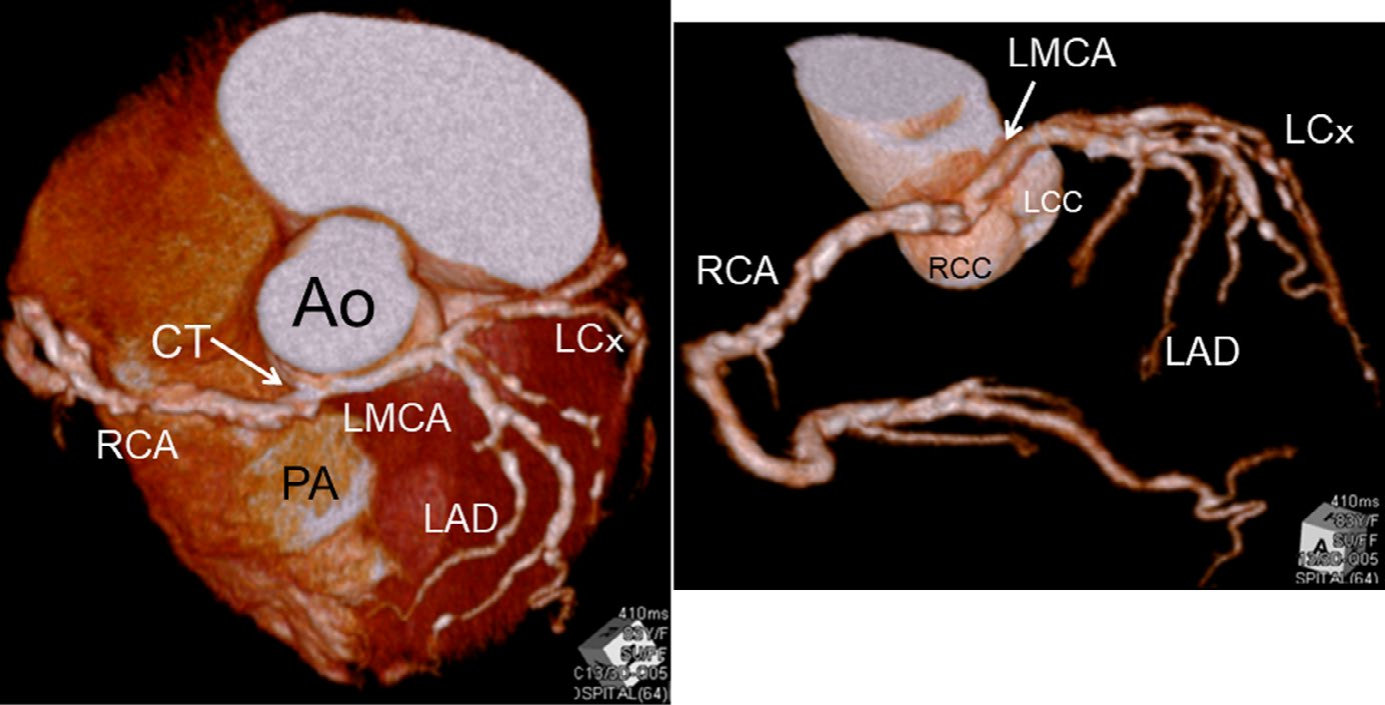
Cardiac multidetector‐row computed tomography findings. Coronary arteries arise from single ostium at right sinus of Valsalva, and left main coronary artery runs between aorta and pulmonary artery. Ao, Ascending aorta; CT, short common trunk; LAD, left anterior descending coronary artery; LCC, left coronary cusp; LCx, Left circumflex artery; LMCA, left main coronary artery; PA, pulmonary artery; RCA, right coronary artery; RCC, right coronary cusp
